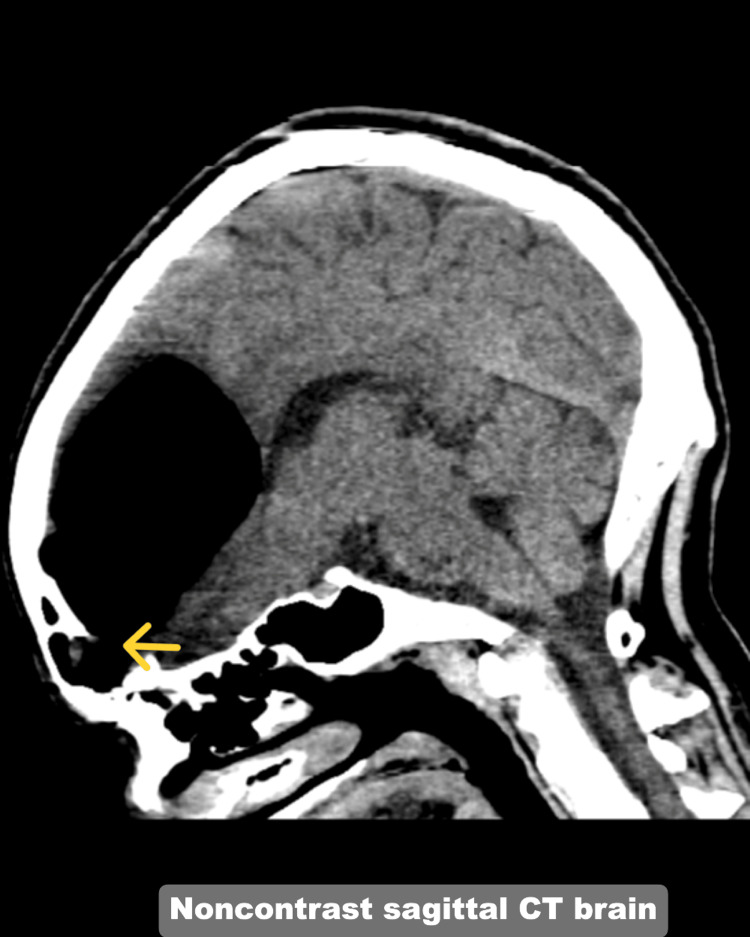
Day 40 non-contrast sagittal CT brain showing large intracerebral pneumatocele communicating with the right frontal sinus Yellow arrow demonstrates complete resolution of the right frontal hemosinus, establishing intracranial communication with resultant formation of a large intracerebral pneumatocele.
